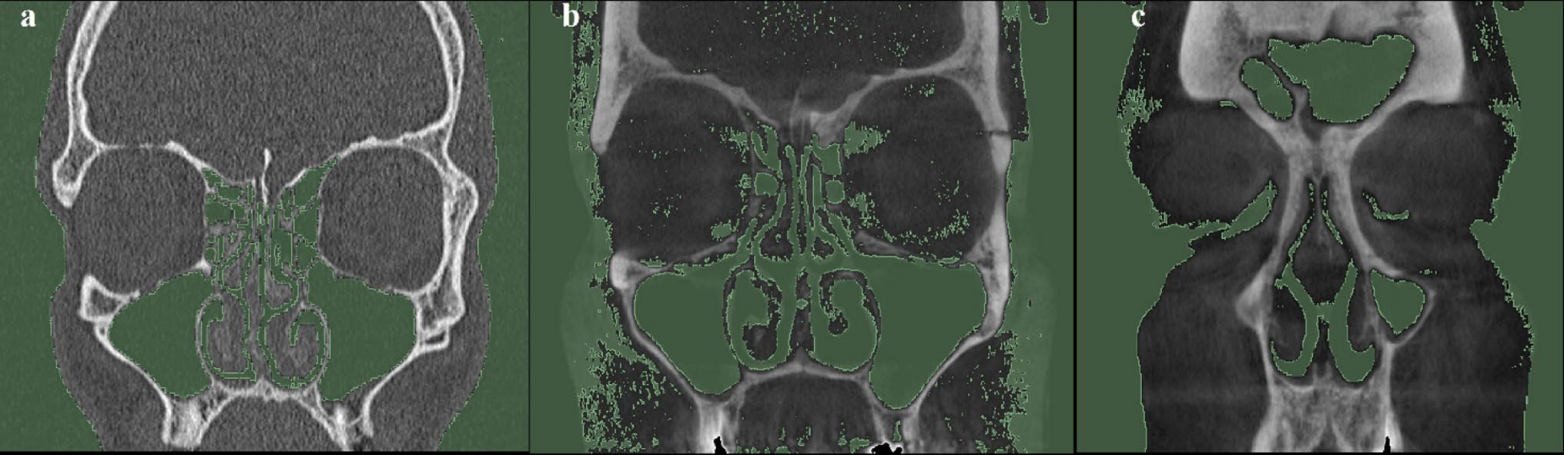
Comparison of the image during the isolation of air within the upper respiratory tract based on a CT (Threshold level min. − 1024 HU, max. − 300 HU) and b CBCT (Threshold level min. − 1024 HU, max. − 300 HU) c CBCT (Threshold level min. − 1024 HU, max. − 600 HU)
